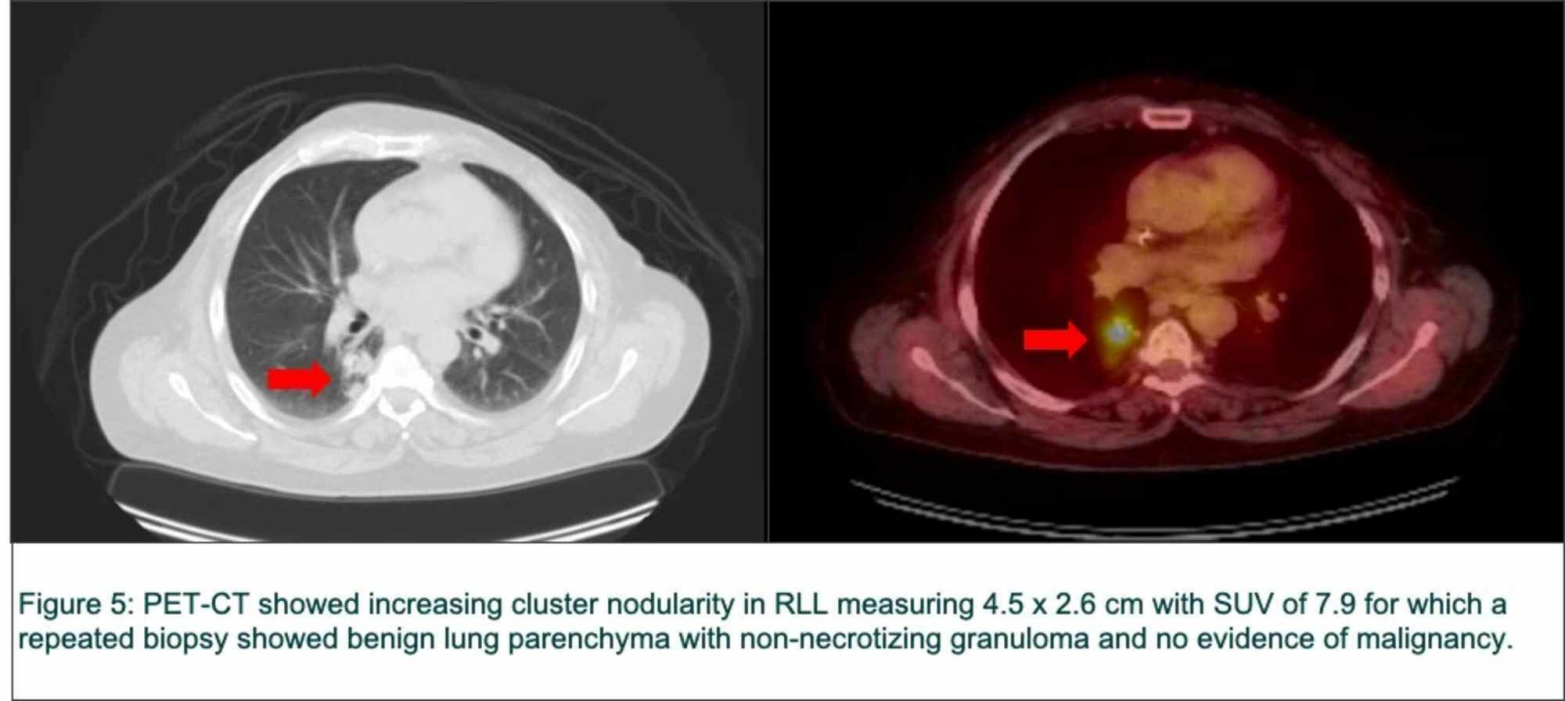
Positron emission tomography-computed tomography (PET-CT) images of the lung RLL: right lower lobe; SUV: standard uptake value.
